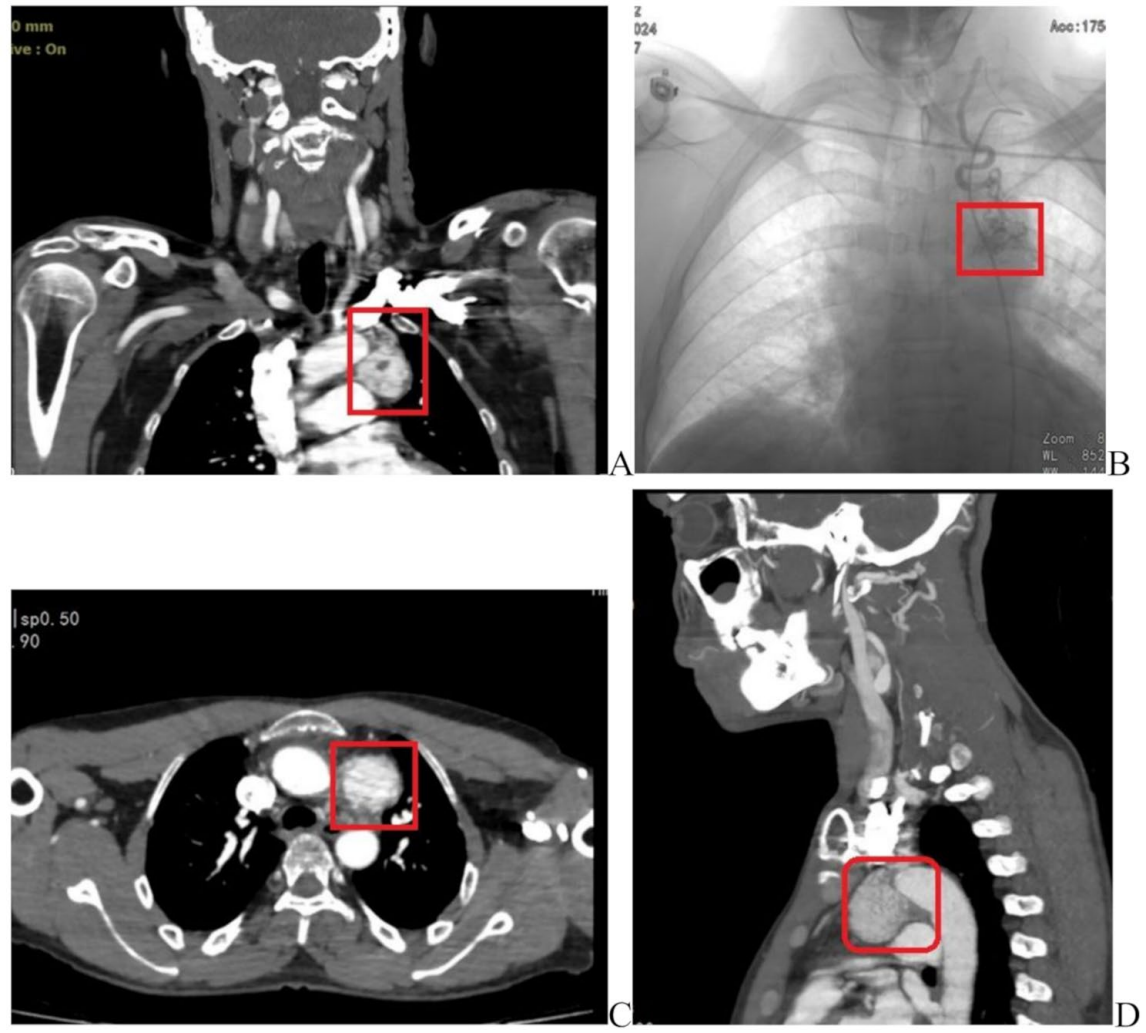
CT angiography of the aorta showing a mass in the aortopulmonary prevascular space
